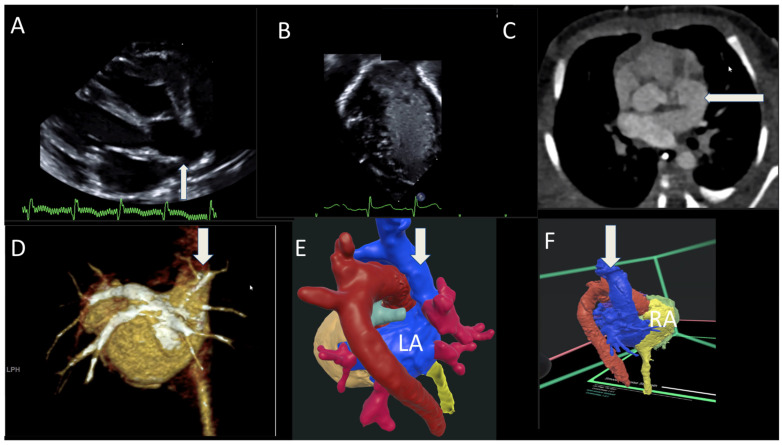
(A,B) TTE 2D and bubble contrast of the SVC connection to the LA arrow; (C,D) CT chest showing SVC draining into the LA; (E,F) VR showing the SVC anomalous draining into the LA. RA—right atrium. LA—left atrium.
